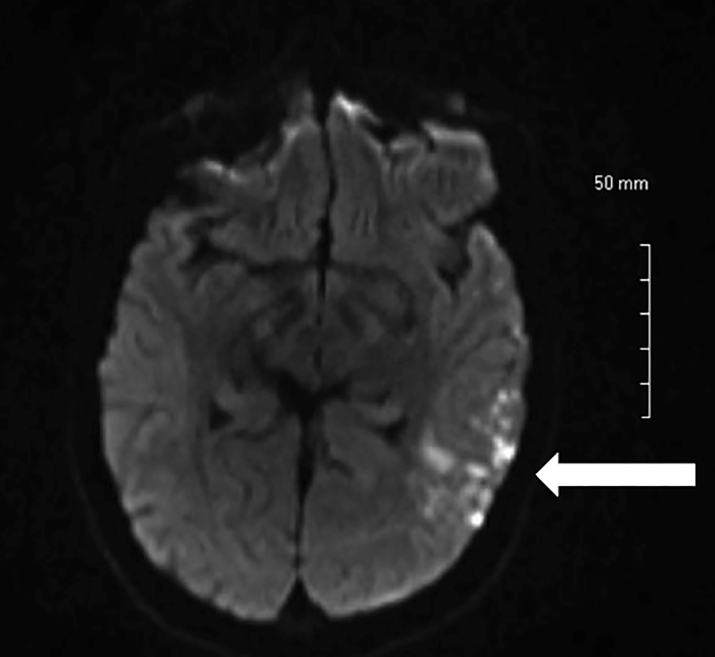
MRI of the brain showing multiple foci of subacute infarcts. *MRI,* Magnetic resonance imaging
